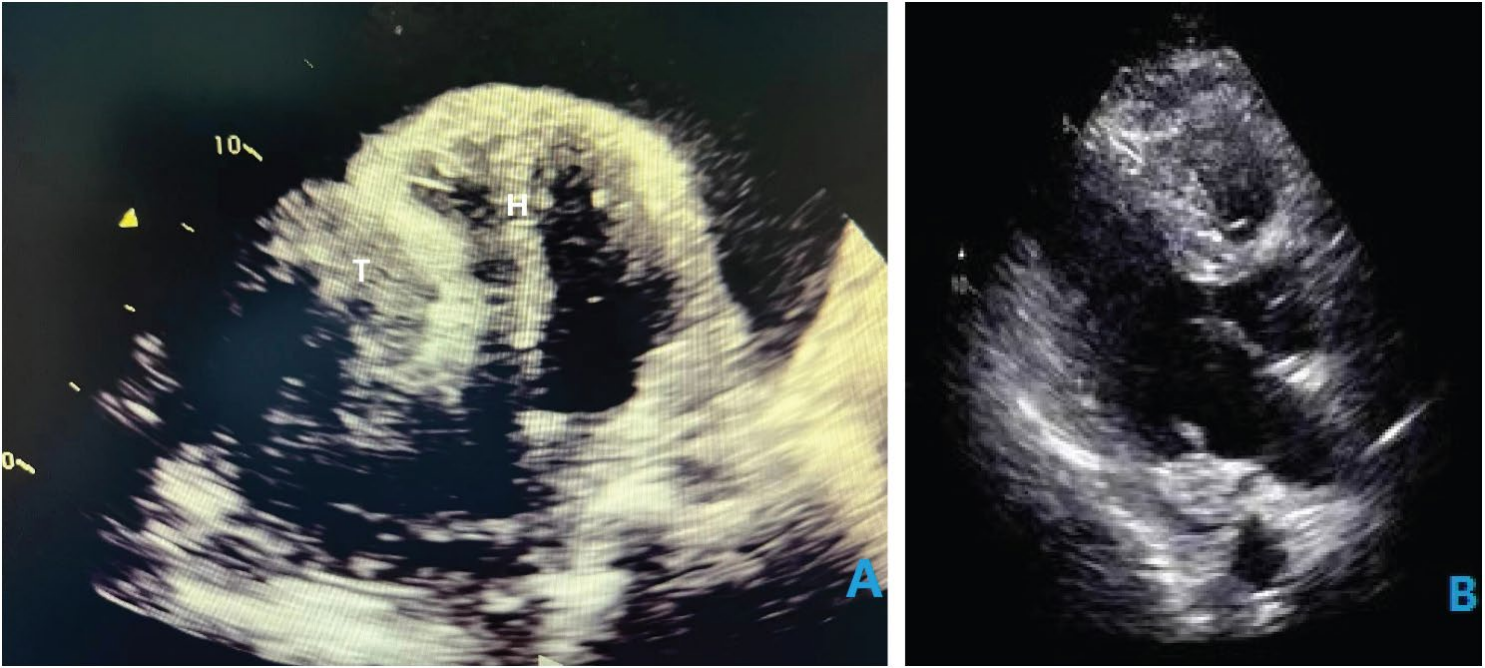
(A) Echocardiography showing intrapericardial space-occupying lesions. (B) Post-operative echocardiography. T: tumor; H: heart
